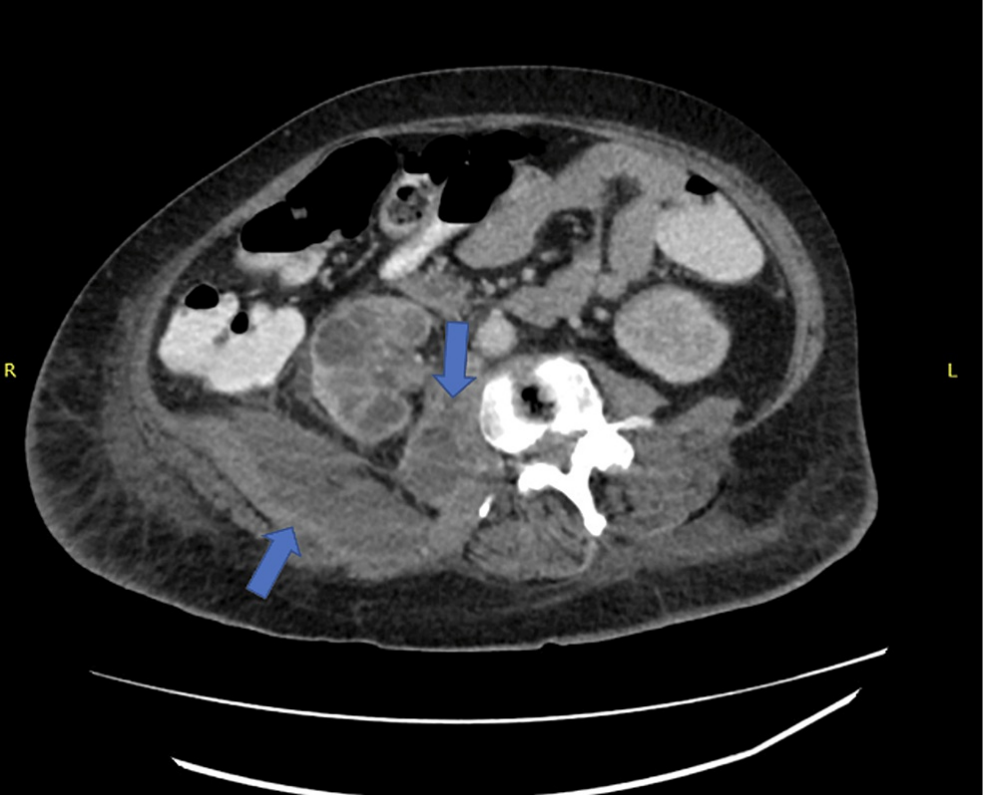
Initial computed tomography demonstrating evidence of a psoas abscess with a large fluid collection around the psoas muscle and retroperitoneal tissues (arrows)
